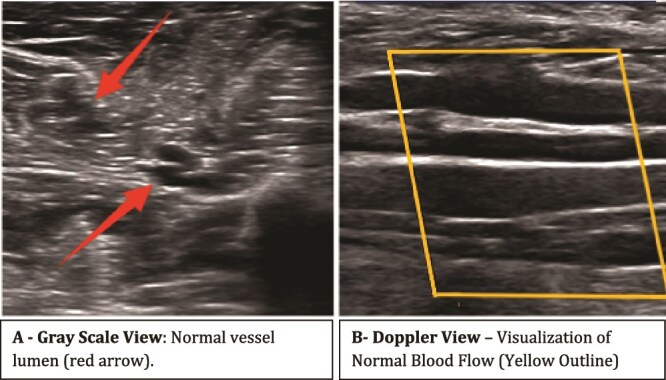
Doppler ultrasound of right leg vessels.
